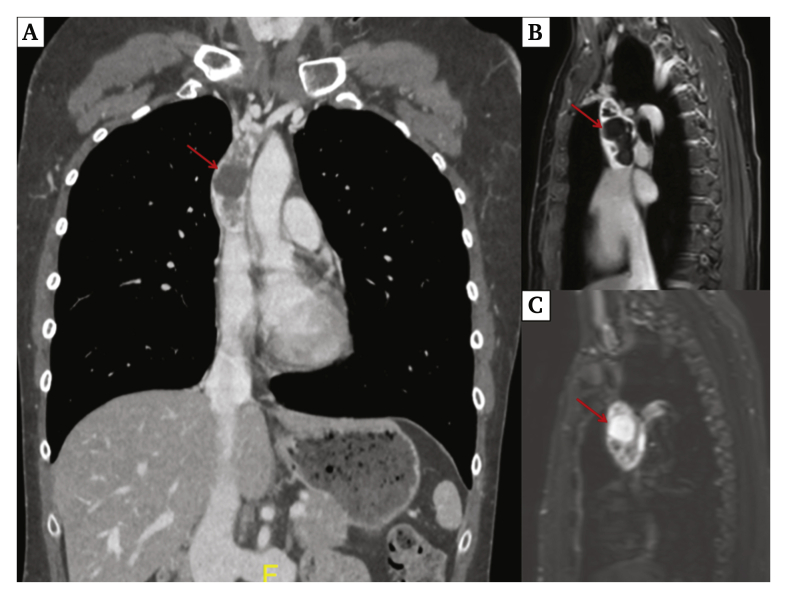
(A) Thoracic computed tomography angiography, coronal reconstruction showing the tumour (red arrow) filling the superior vena cava lumen. (B) Thoracic magnetic resonance imaging T2 weighted, and (C) T1 weighted with gadolinium injection.
